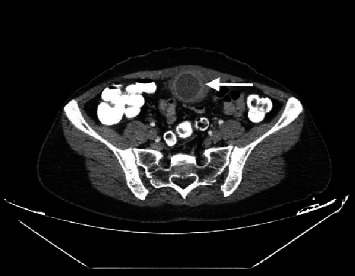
Contrast–enhanced computed tomographic image showing a cystic lesion with peripheral enhancement (arrow), thus excluding the initial diagnosis of an incarcerated hernia.
